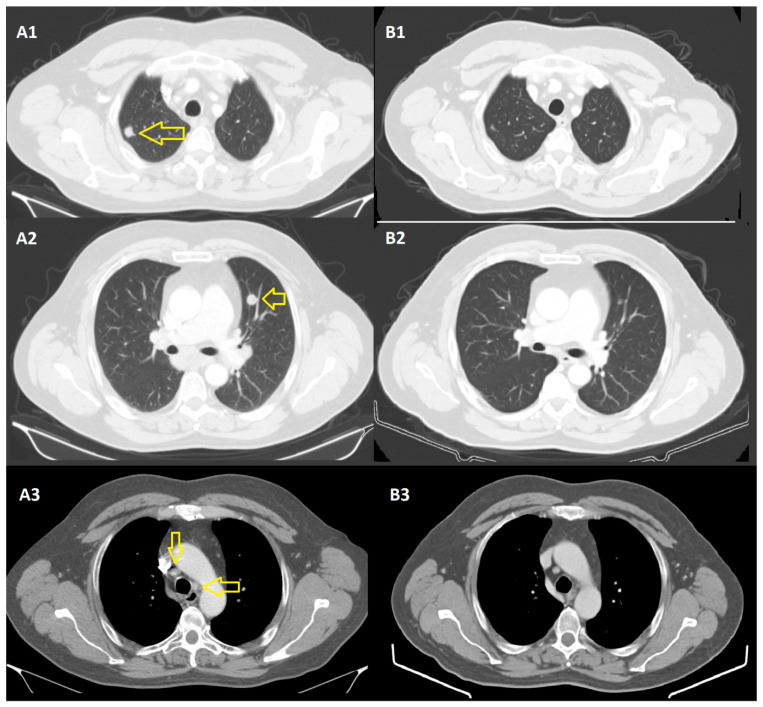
CT scans from December 2020 (A1–A3), compared to those from June 2021 (images (B1–B3)), where pulmonary metastases (yellow arrows) and adenopathies are in regression.
